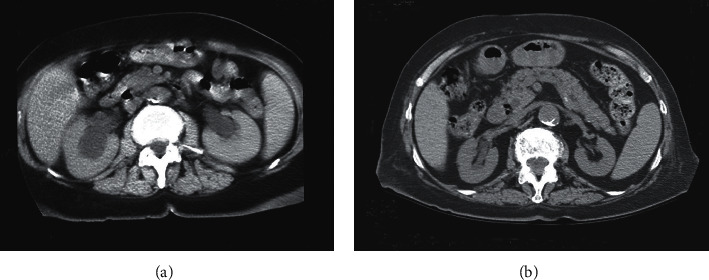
Native CT scan (a) at admission showing massive bilateral hydronephrosis and (b) at 3 months showing a complete regression of the hydronephrosis on the left side and a partial regression on the right side.
